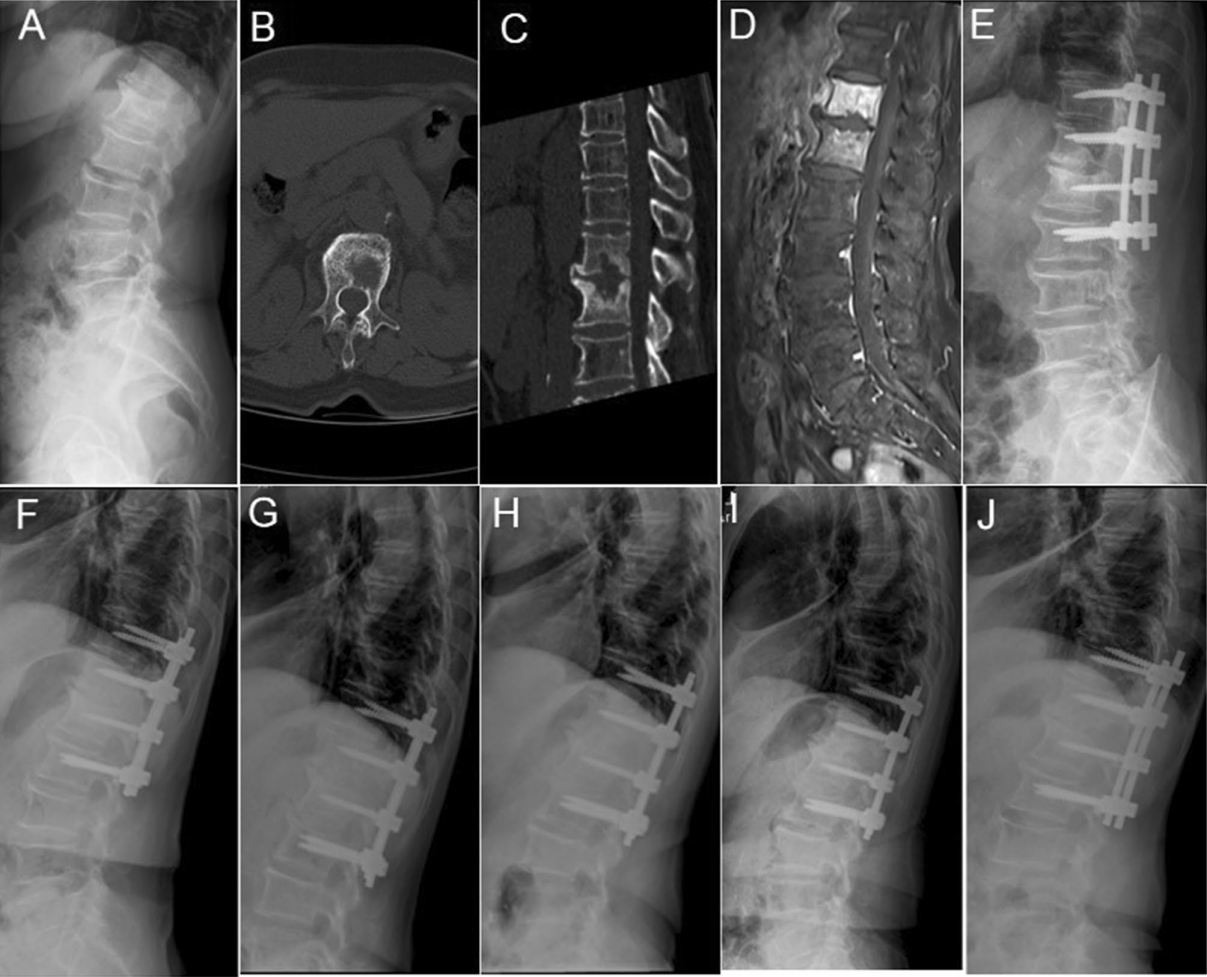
An X-ray revealed T12-L1 disc space narrowing with end-plate destruction (a); Computed tomography and magnetic resonance imaging (b–d) show vertebral bone destruction and paravertebral abscess formation. X-rays taken at 3 days, 3 months, 6 months, 1 year, and 2 years post-operatively, and at the final follow up, respectively (e–j).
